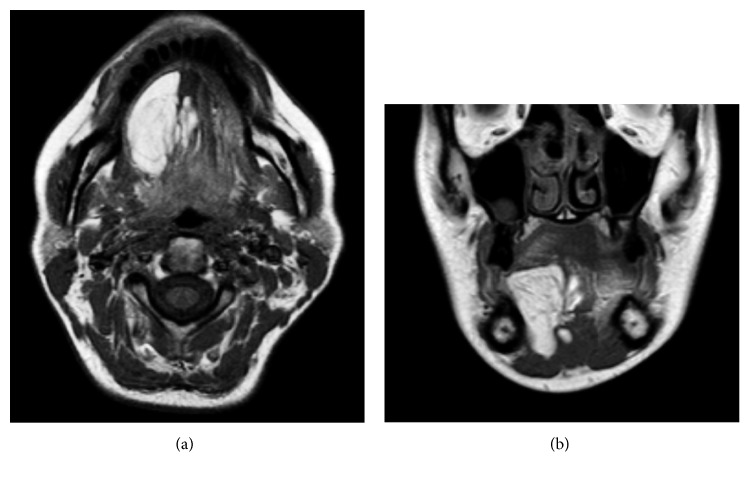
Axial (a) and coronal (b) MRI of the lesion (see text for description).
